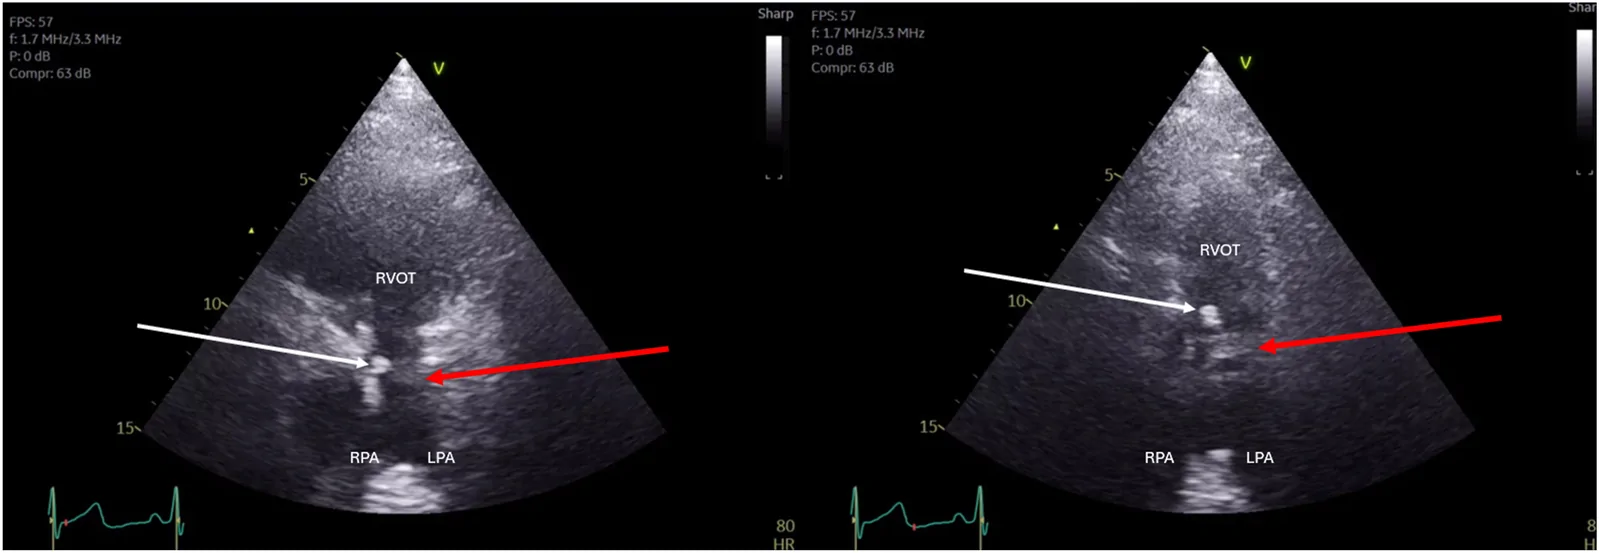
Two-dimensional TTE, basal parasternal short-axis systolic *(left)* and diastolic *(right)* views at the level of the RVOT, demonstrates a mobile echogenic mass *(white arrow)* attached to the PV *(red arrow)*, consistent with a vegetation. *LPA*, Left pulmonary artery; *RPA*, right pulmonary artery.
